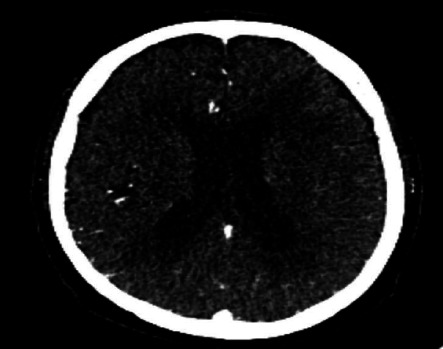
CT scan of the brain done after a year of treatment showing a marked decreased in vasogenic oedema and malignant changes.
